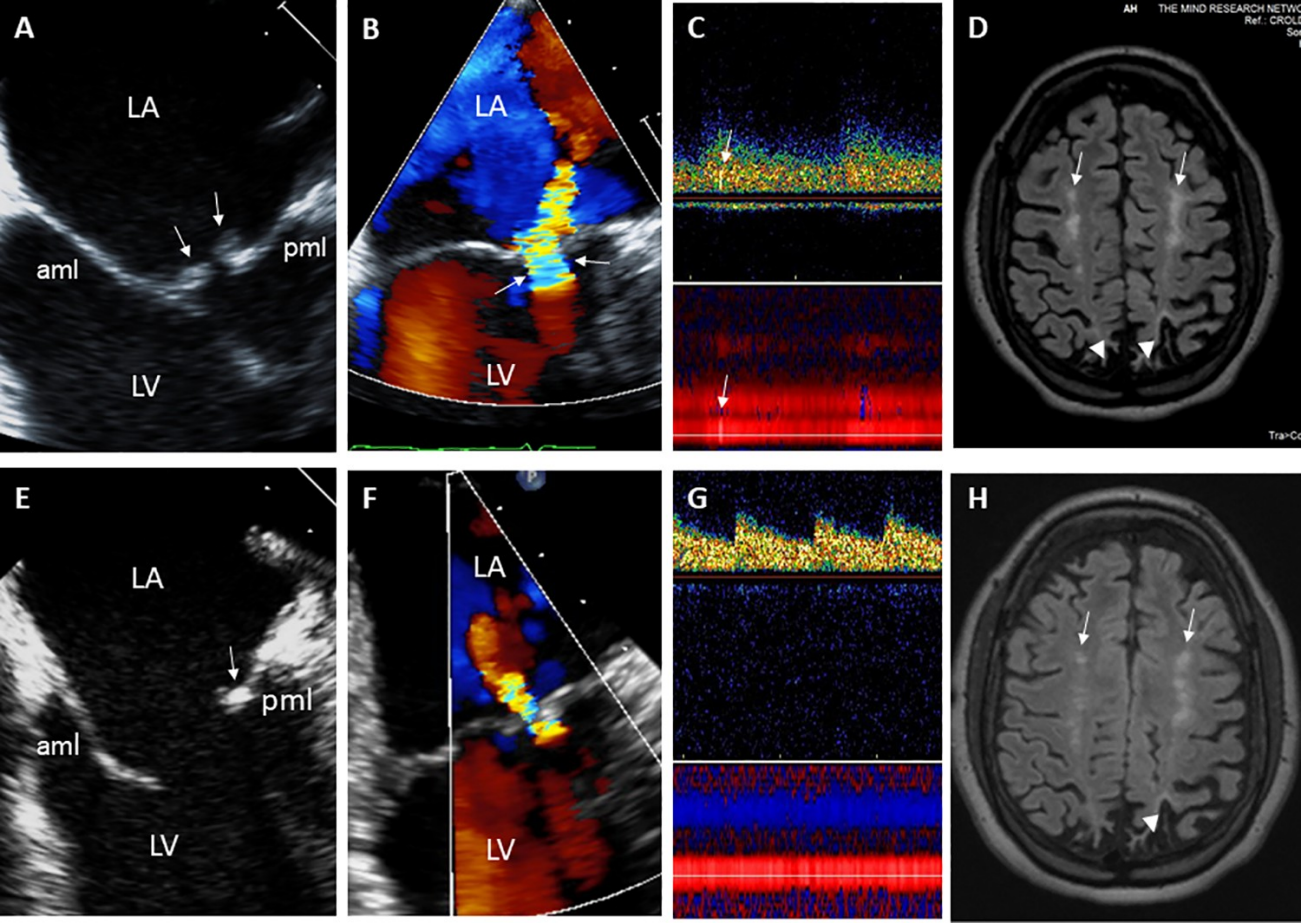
21-year-old female with Libman-Sacks Endocarditis complicated with acute transient ischemic attack and previous stroke. A,B. These 2D and color-Doppler TEE views demonstrate a moderate size (area 0.85 cm^2^), sessile, and oval shaped Libman-Sacks vegetations (arrows) on the distal portions and atrial side of the anterior (aml) and posterior (pml) mitral leaflets (A) and moderate to severe eccentric mitral regurgitation as demonstrated by a large flow convergence zone (arrows) (B). C. Transcranial Doppler of the left middle cerebral artery demonstrates 1 of 4 microemboli (arrows). D. On MRI, cerebral infarcts (arrowheads) and multiple white matter lesions (arrows) for a lesion load of 7.05 cm^3^ were demonstrated. Her global neurocognitive dysfunction was graded as severe. E,F. After 3 months of warfarin, hydroxychloroquine, prednisone, and mycophenolate mofetil, repeat imaging demonstrated resolution of the anterior mitral valve vegetation and reduction in size (arrow) of the posterior mitral leaflet vegetation (E), reduction of mitral regurgitation to mild degree (F), resolution of cerebromicroembolism (G), and decrease in count and size of white matter lesions (arrows in H) to a lesion load of 4.2 cm^3^, and improvement in her global neurocognitive dysfunction to mild degree.
